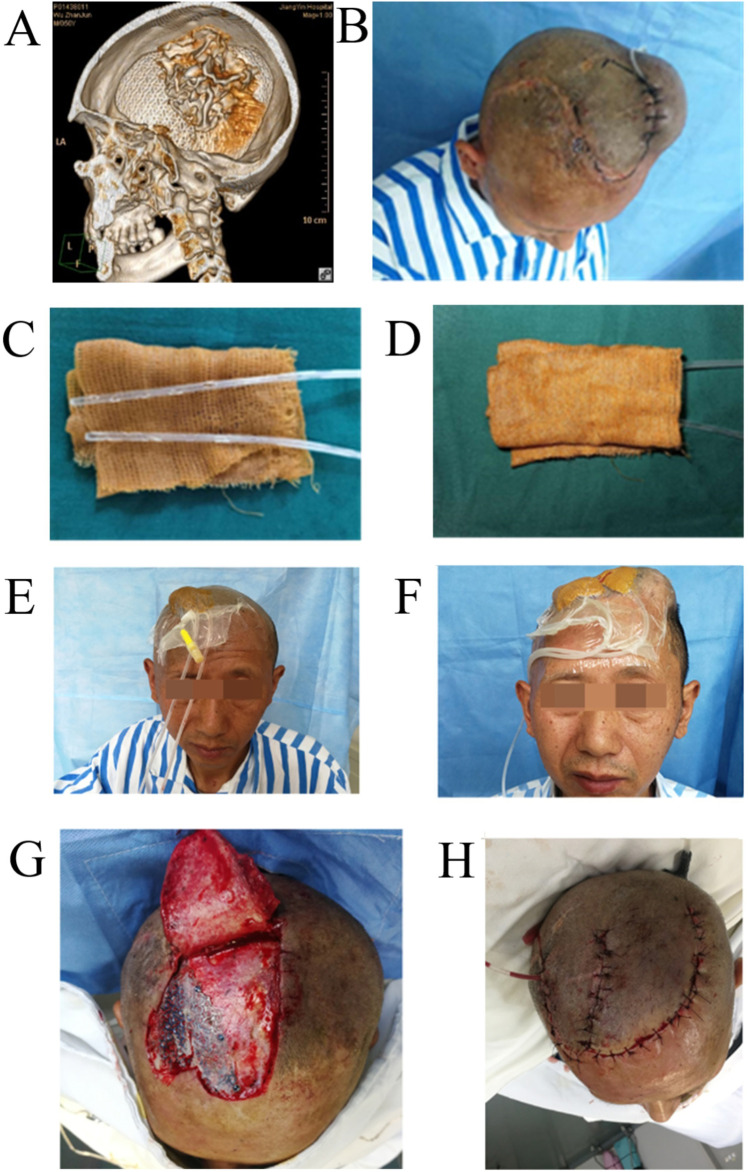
The therapeutic effects of keeping the titanium mesh for exposed titanium mesh after cranioplasty. A Computed tomography (CT) 3D reconstruction showing an infection between the titanium mesh and the dura. B The skin soft tissue expander was embedded in the loose connective tissue layer beneath the cap aponeurosis 3–6 cm from the exposed edge of the titanium mesh. C–D Self-made negative pressure wound therapy materials: silver ion dressing wrapped side wall hole disposable sputum suction tube. E Negative pressure wound therapy was performed for the exposed titanium mesh. A cannula was inserted between the titanium mesh and the dura mater. F Soft tissue expander inserted around the exposed titanium mesh, and negative pressure drainage was continued at the lesion. G Scalp transposition after dilation to repair the exposed titanium mesh. H An adjacent skin flap was taken to repair the defect at the donor area
